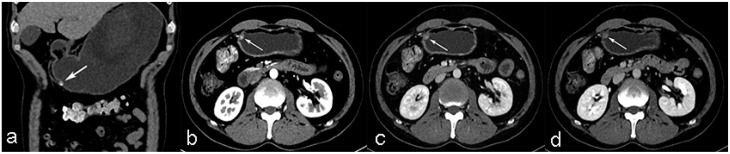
Gastric calcifying fibrous tumor (CFT) a. unenhanced phase coronal CT image shows an elliptical, soft tissue lesion in antrum of stomach with moderate calcification (arrow). b, c and d, arterial phase, portal venous phase and equilibrium phase of CT images show moderate enhancement
